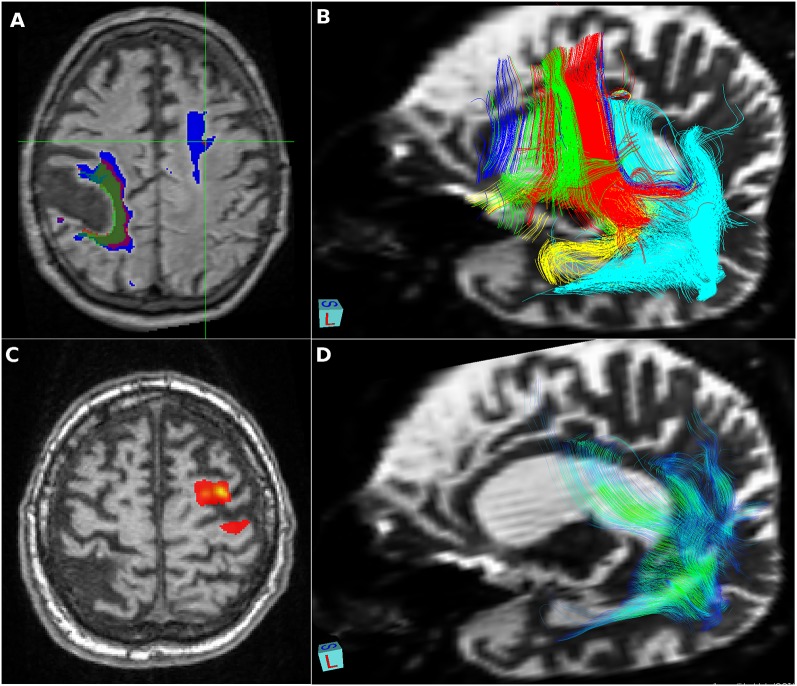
Vascular lesions and tractography—visualization provided using FSLeyes, part of FSL, and deterministic tractographies processed in MedINRIA 1.9.2. (A) Relative increase in size of a right-sided residual poststroke lesion (2010 green, 2012 red, and 2014 blue) and significant growth of the fluid-attenuated inversion recovery (FLAIR) left frontal white matter hyperintensity detected in 2012; (B) deterministic tractography of the dorsal stream: fibers originating from Brodmann’s areas BA44 in red, BA45 in green, inferior frontal gyrus in blue, insula in yellow, superior temporal gyrus in turquoise, and precentral gyrus in violet; (C) probabilistic tractography shows only local connectivity to the precentral gyrus; (D) deterministic tractography of the ventral stream: fibers originating from the angular gyrus in color reflecting the value of local fractional anisotropy.
